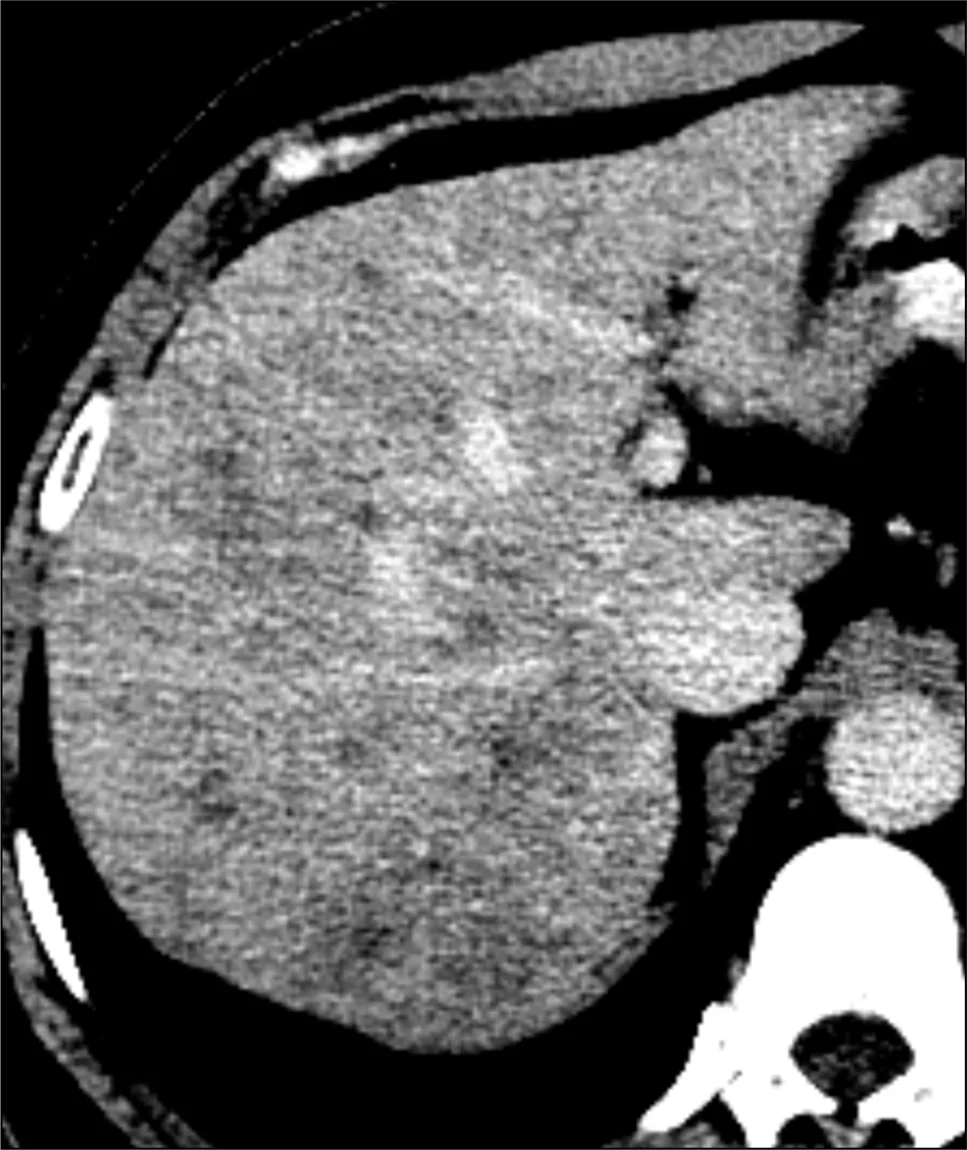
Contrast-enhanced axial CT images of the abdomen showing diffuse heterogeneous hepatic parenchyma with numerous small hypodense hypoenhancing nodules that led to further evaluation by MRI. CT, computed tomography.
